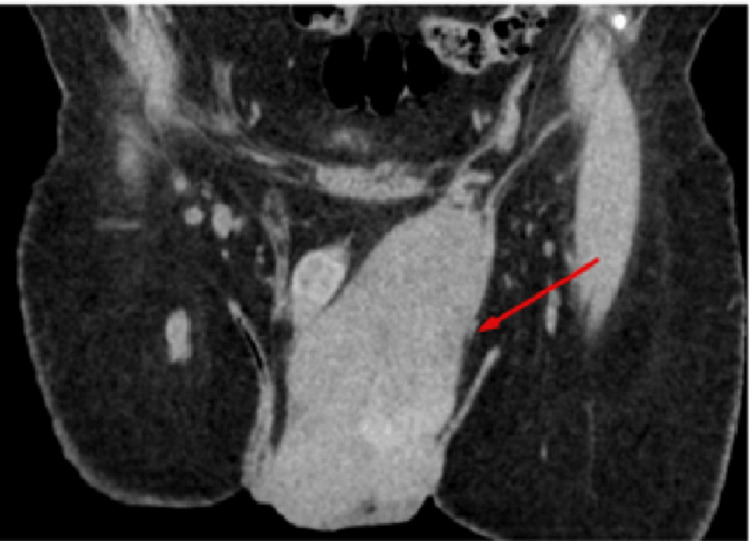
Coronal section of non-contrast CT shows its extension from left inguinal region to left hemiscrotum (red arrow).
